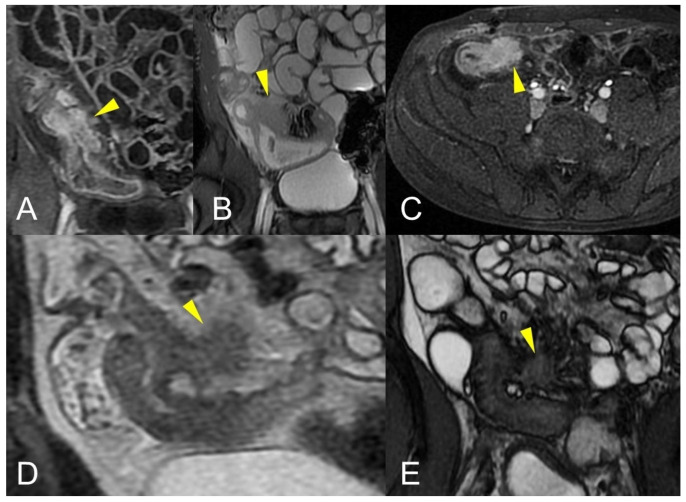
Inflammatory conglomerate represents a climbing mesenteric fat involvement in the context of penetrating disease and mesenteric inflammation. The components of the fistulas within the mass can be difficult to detect because it often coexists with phenomena of retraction that are expressed on the intestinal loops nearby or on the ureters. Small inflammatory conglomerate in the context of the mesentery adherent to a segment of the pathological small intestine, with active disease (yellow arrowheads in (A,C): coronal (A) and axial (C) contrast-enhanced fat-suppressed T1-weighted image and in (B,E) (coronal fast imaging employing steady-state acquisition; FIESTA image) and (D) (coronal T2-weighted MRE image)).
